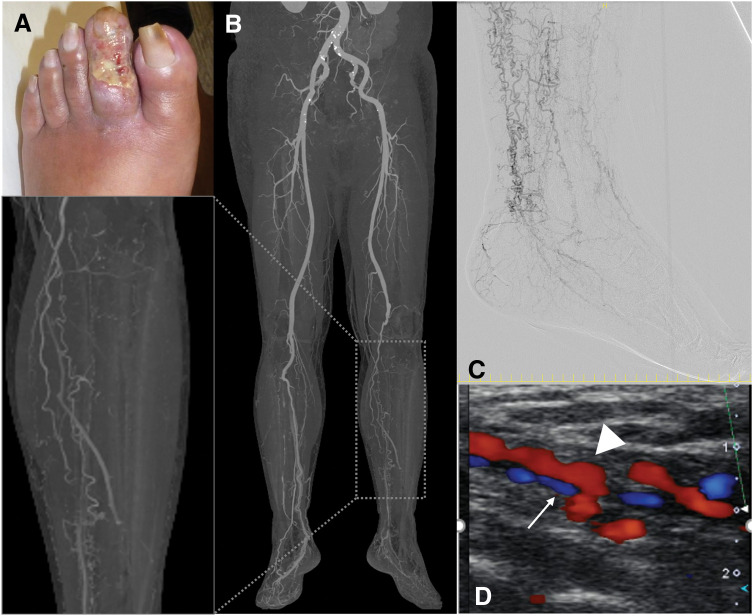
Clinical presentation and preoperative evaluation. Left 2nd toe ulcer observed at the time of admission (A). CTA demonstrated occlusion distal to the left popliteal artery, with foot perfusion maintained via collateral circulation (B). Digital subtraction angiography revealed the absence of normal arteries in the foot, with the presence of corkscrew-like collateral vessels (C). Preoperative ultrasonography confirmed the patency of the plantar arteries and veins. The arrow indicates the plantar artery, and the arrowhead indicates the plantar vein. Despite the small vessel diameters, a potential site for anastomosis was identified (D).
